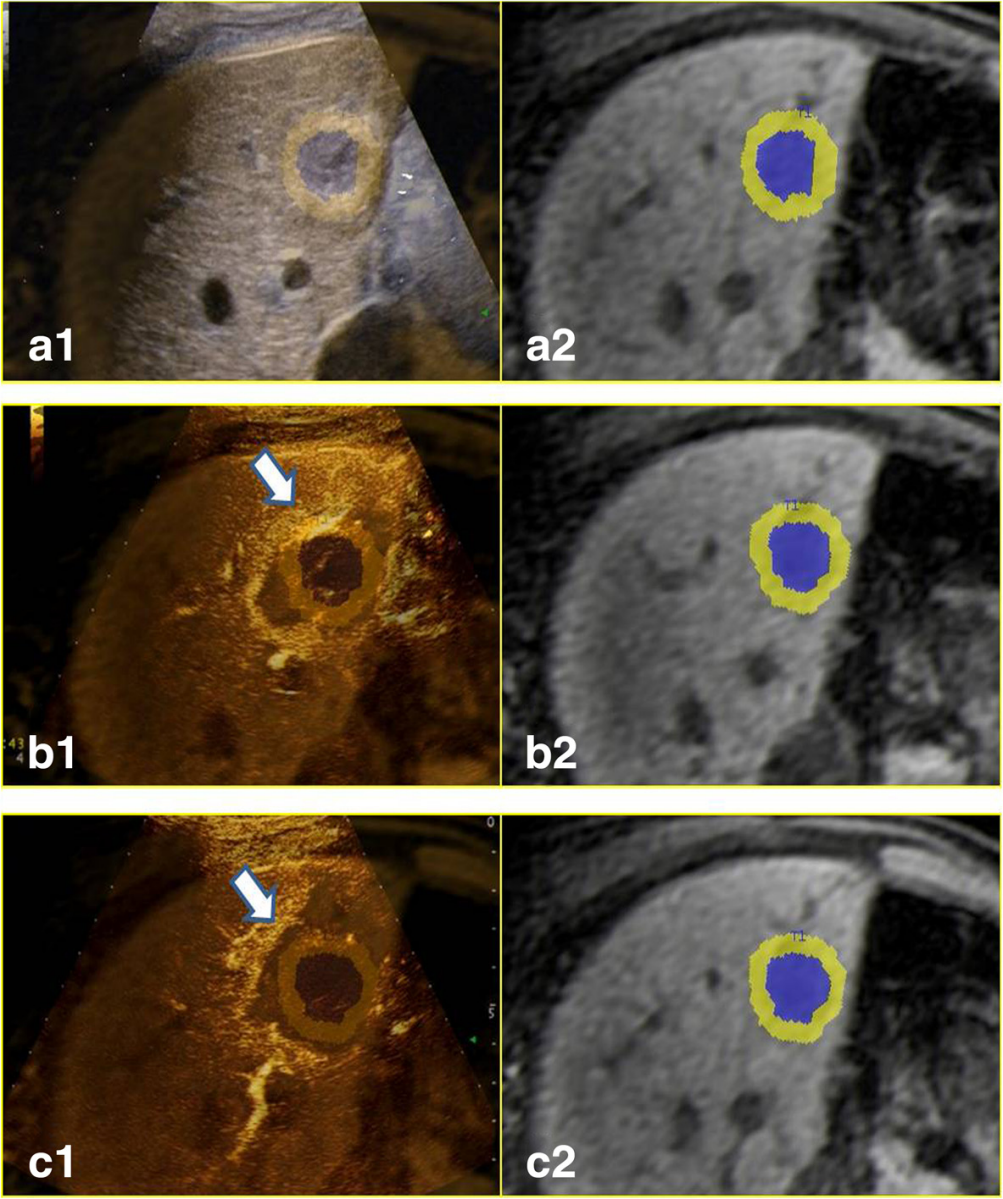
a1 and a2 For the lesion in Fig. 2, ultrasound image was fused with the MR image; a1 shows the overlapped ultrasound and MR image with the tumor in blue and the AM in yellow, and a2 shows the MR image with the tumor outlined and the AM. After ablation, CEUS-MR image fusion showed an inadequate AM (*arrow* in b1); b2 shows the MR image with the tumor outlined and the AM. After supplementary ablation, the inadequate AM became an adequate AM (*arrow* in c1); c2 shows the MR image with the tumor outlined and the AM
